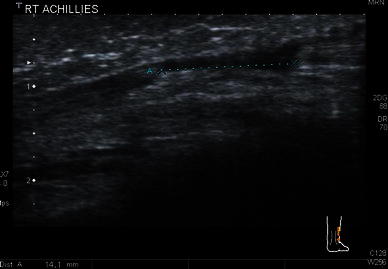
Ultrasound measurement of the tendon gap after Achilles tenotomy with the foot in a neutral position
